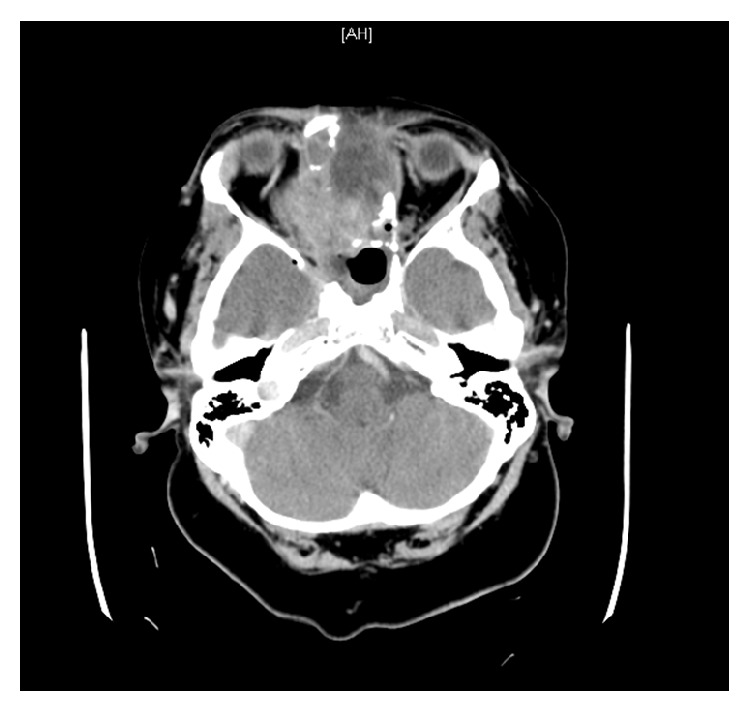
Noncontrasted CT demonstrating tumor extension.
